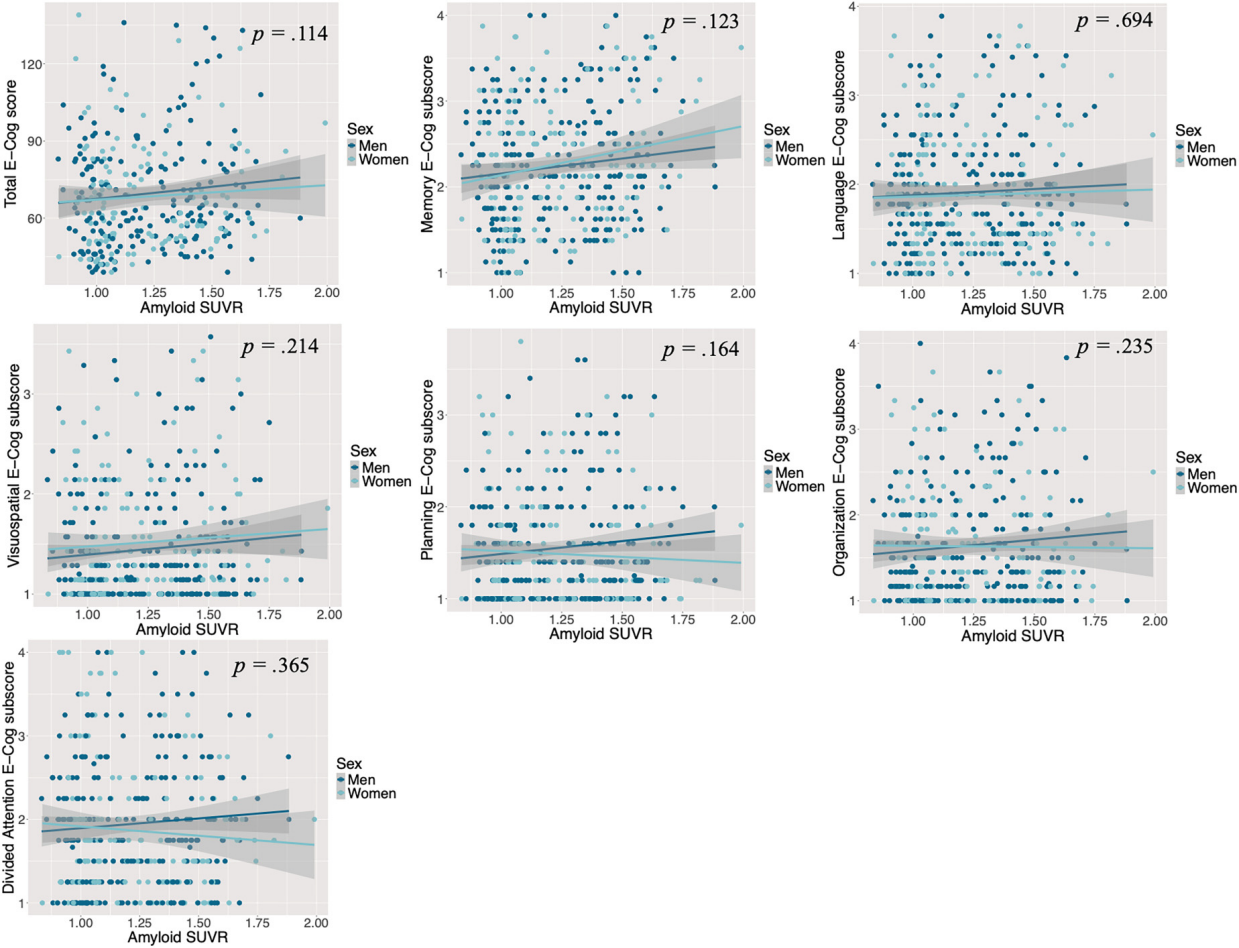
The association between Aβ levels and the severity of cognitive complaints, by sex and domain, controlling for age, years of education and symptoms of depression and anxiety in individuals at the MCI stage. Reported *p*values reflect the associations between Aβ levels and E-Cog scores for the whole MCI sample (men and women combined). Here, Aβ levels were not associated with E-Cog scores in any cognitive domain in MCI individuals. As no significant sex interaction was detected, corresponding *p*values are not presented. E-Cog: Everyday Cognition Questionnaire; MCI: Mild Cognitive Impairment; SUVR: Standardized Uptake Value Ratio.
